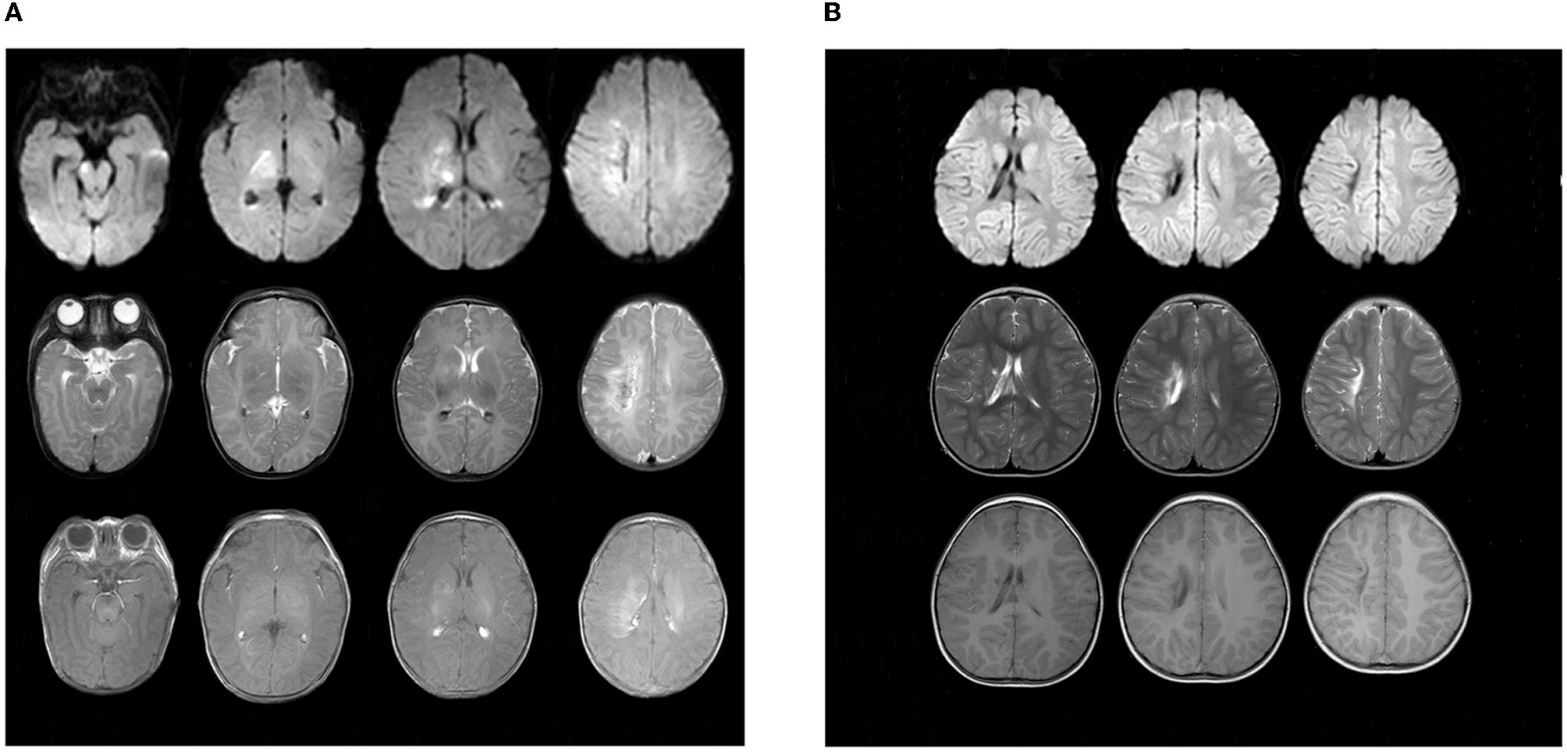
Findings in sequential MRI brain scans. (A) was taken upon admission in October 2018, showing multiple spots of white matter hyperintensities (in the right central semiovale, lateral ventricle, and basal ganglia) with focal hemorrhage. Patchy, limited diffuse, high signal intensity can be seen in the right central semiovale, lateral ventricle, basal ganglia, and the right side of the brain stem in diffusion-weighted imaging (DWI). (B) Obtained in March 2021, there were still multiple cerebral injuries with enlarged encephalomalacia in the right centrum semiovale, lateral ventricle, and basal ganglia compared with those seen in the previous scan.
